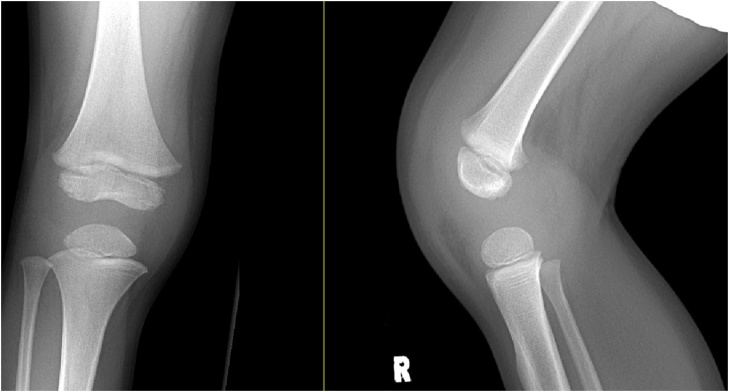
Radiographs taken of the right knee the following day upon re-evaluation. An effusion can be appreciated along with soft tissue swelling without apparent osseous involvement.
